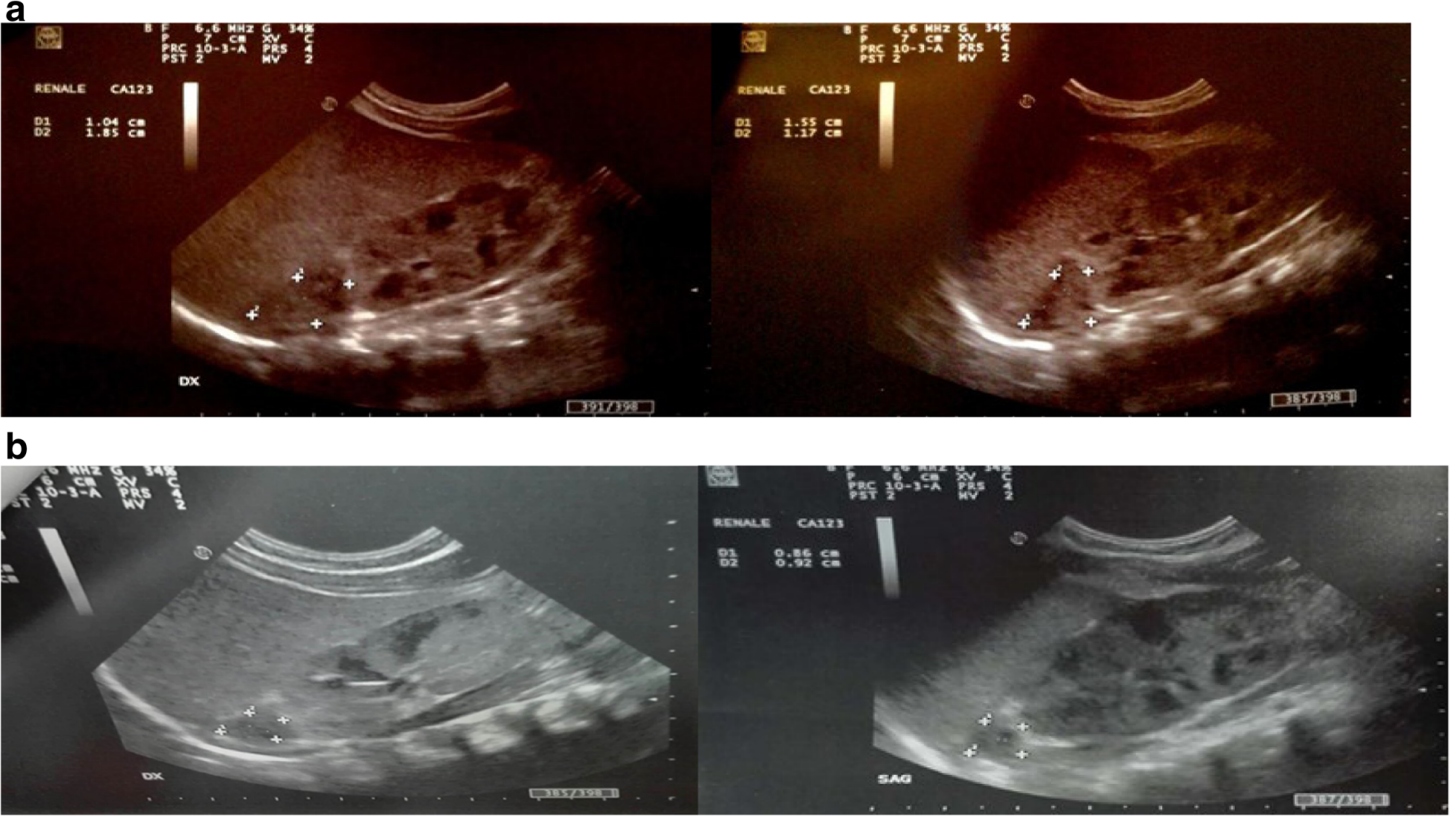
Progressive ultrasound reduction of lesions at 45 days of life (a) and at 60 days of life (b)
